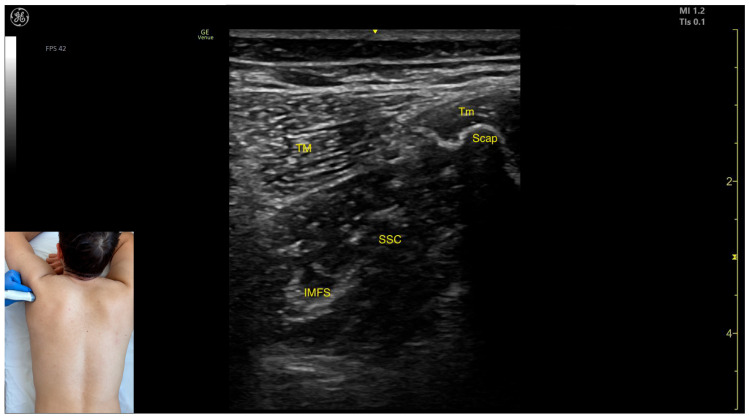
Ultrasound anatomy of the subscapularis (SSC) posterior view with key landmarks: SSC—subscapularis; TM—teres major; Tm—teres minor; Scap—scapula; and IMFS—intramuscular fascial septum.
